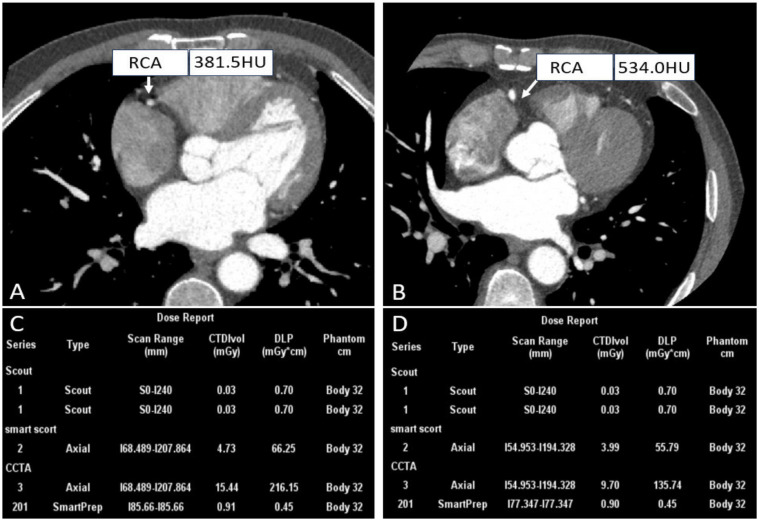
Right coronary CTA images and dose reports of patients with different heart rates. ((A,C) A patient with a heart rate of 228 bpm; (B,D) A patient with a heart rate of 71 bpm).
